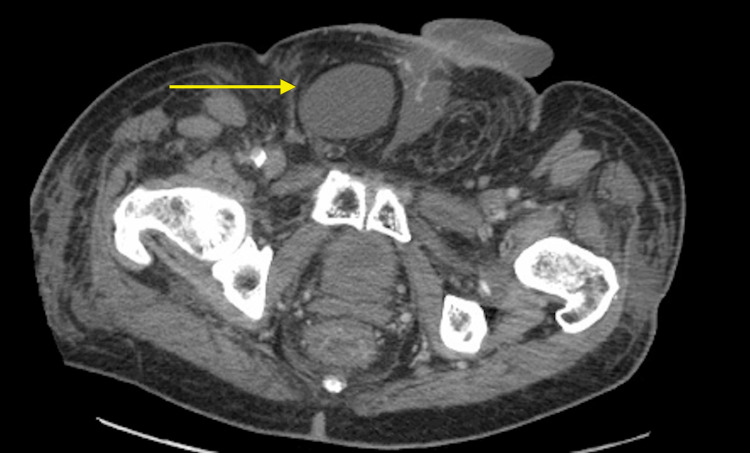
Transverse CT imaging of the patient at the time of penile and scrotal metastatic presentation displaying infiltration into the right hemiscrotum. CT: computed tomography
